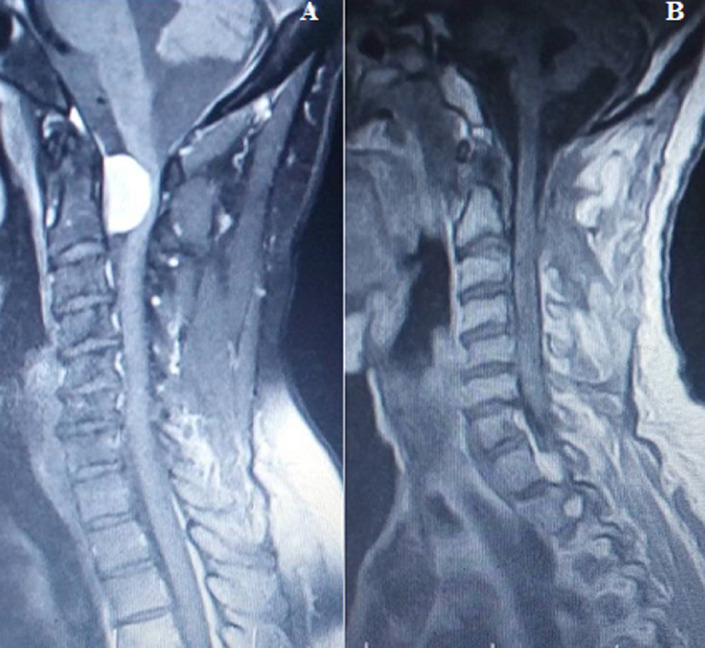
pre (A) and postoperative (B) cervical spine MRI images showing gross total tumour excision in one of our patients
